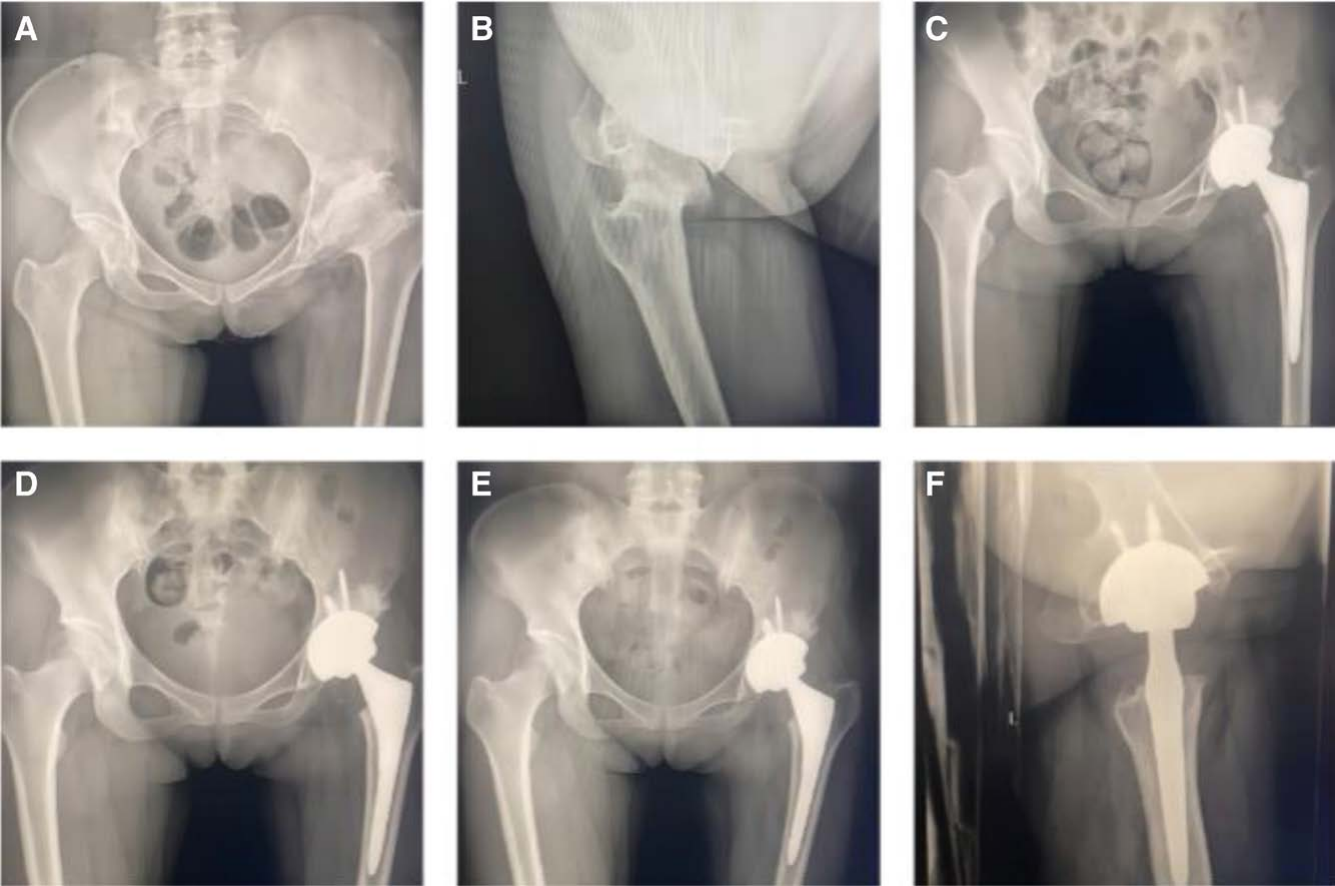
Posterolateral approach total hip arthroplasty for a 37-yr-old female patient. (A) Preoperative pelvis X-ray. (B) Preoperative hip X-ray. (C) Postoperative pelvis X-ray 3 mo after surgery. (D) Postoperative pelvic X-ray 6 mo after surgery. (E) Postoperative pelvic X-ray 1 yr after surgery. (F) Postoperative hip X-ray 1 yr after surgery.
